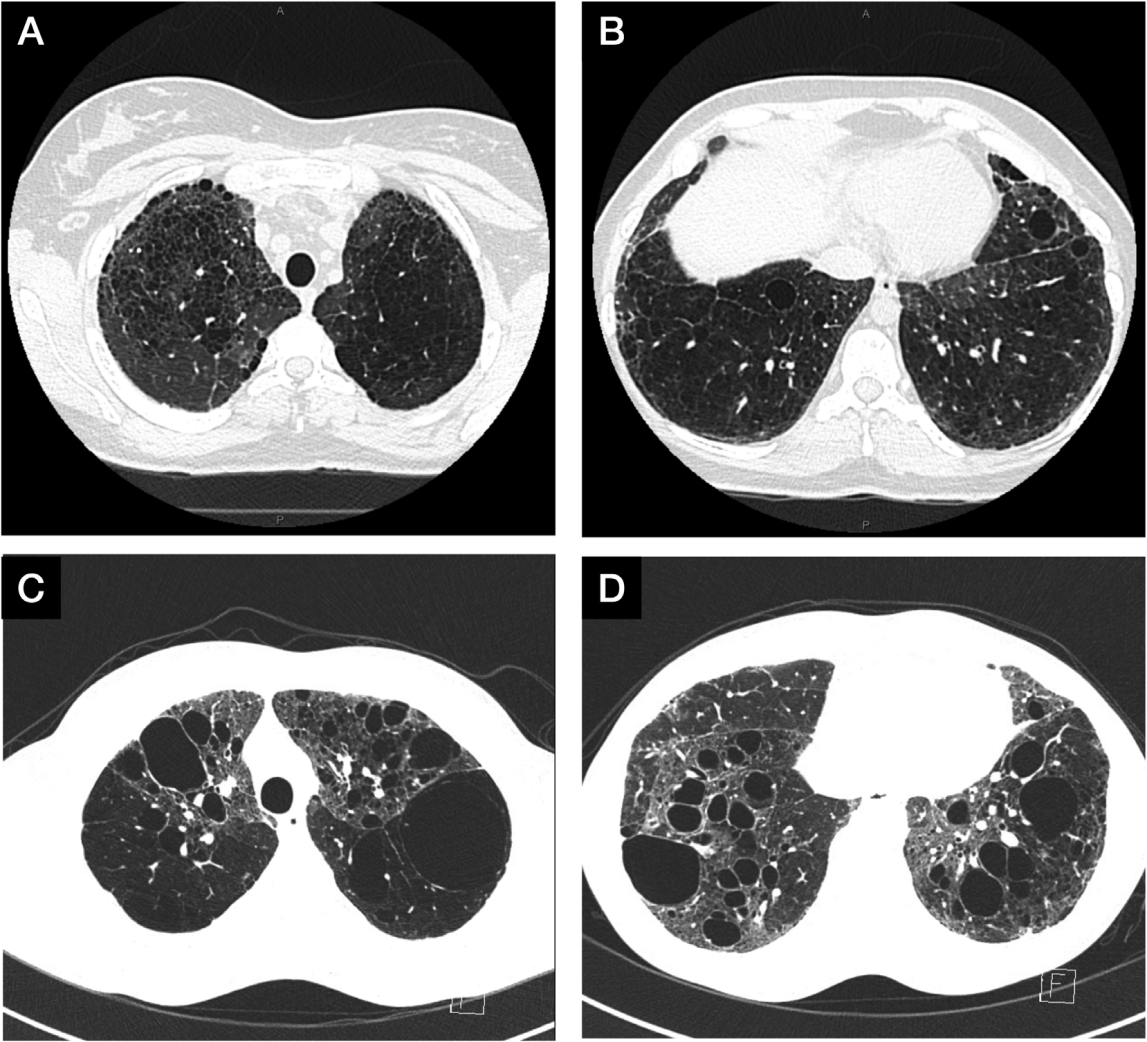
High‐resolution computed tomography (HRCT) scan of the chest for the sister (A and B) and the brother (C and D). (A) HRCT of the chest at age 28 years demonstrating extensive cystic change in the upper lobes including subpleural regions. There is a small area of normal appearing lung in the posterior segment of the right upper lobe. (B) There is extensive vascular attenuation in the lower lobes with multiple well‐defined cysts up to 20 mm in size at the bases. (C and D) HRCT of the chest at age 30.5 years demonstrating extensive thin walled cysts measuring from a few millimetres to 60 mm. There are also areas of reticulation and parenchymal distortion. The cysts are larger and more prominent in the brother than the sister.
